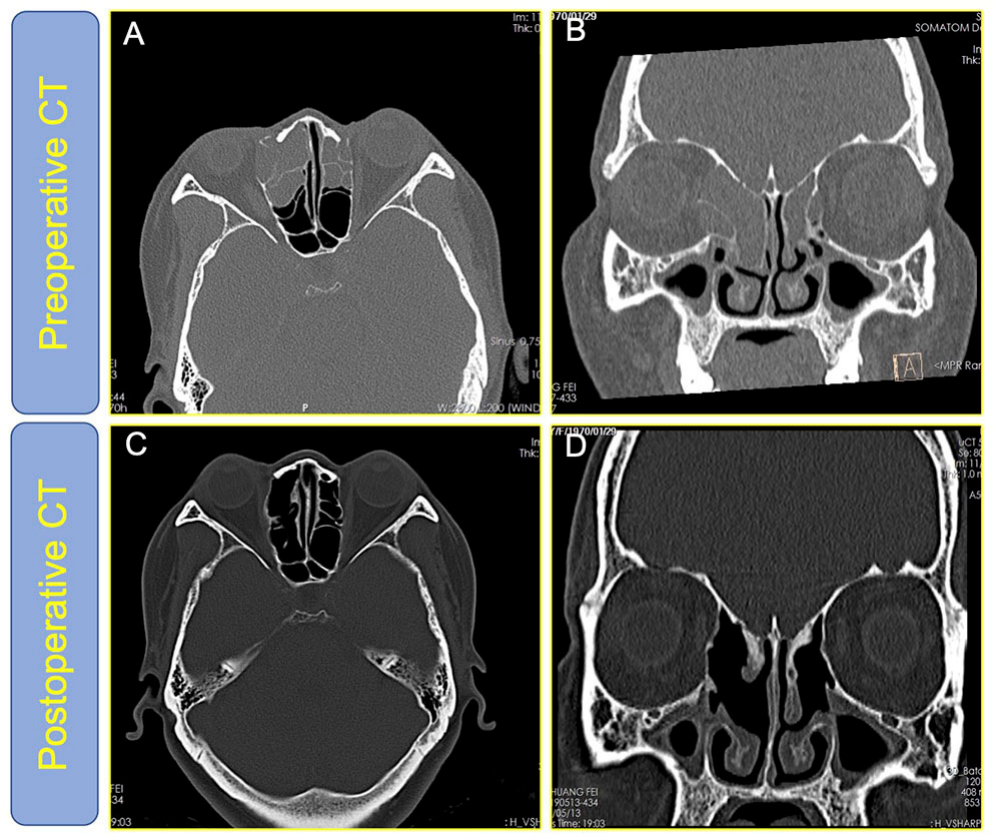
(A,B) The high-resolution CT of the nasal sinuses of patient #70 before surgery; (C,D) The high-resolution CT of the nasal sinuses of patient #70 1 year after surgery showed that all diseased sinuses were open and the disease-free sinuses are undisturbed and the nasal mucosa is well preserved.
